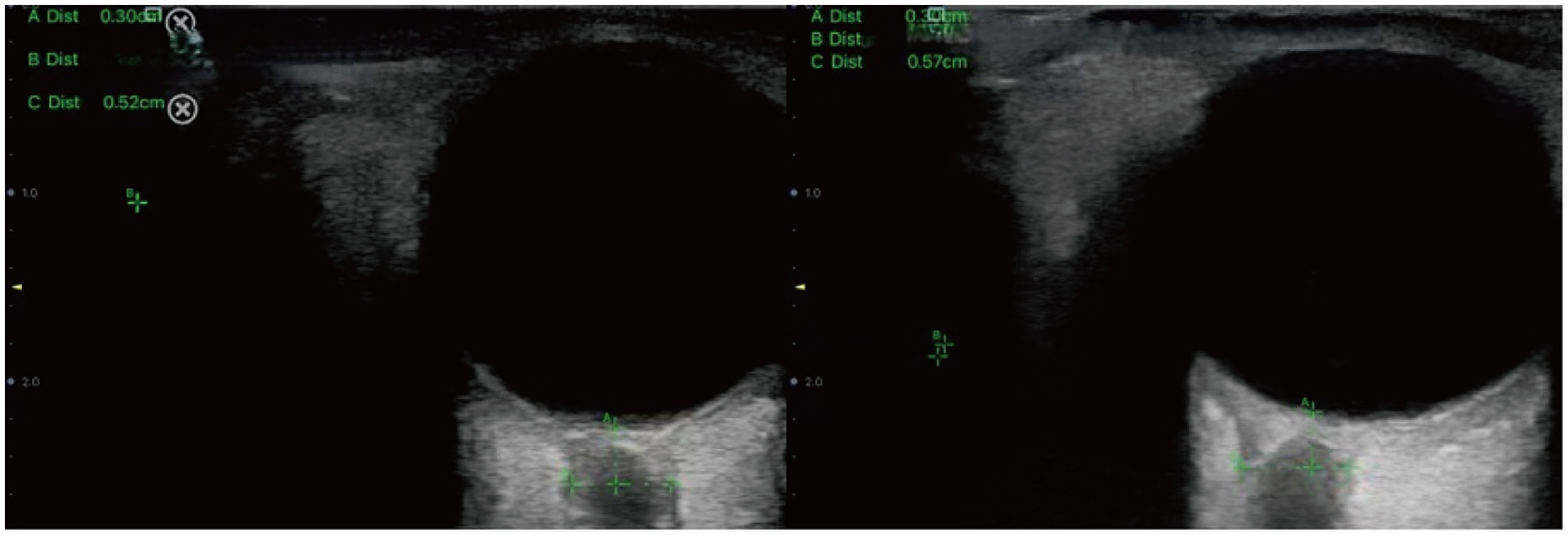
Sonographic measurement of the ONSD 3 mm behind the eyeball. The left ONSD was 0.52 cm in a patient from Group 1, and the right ONSD was 0.57 cm in a patient from Group 2.
